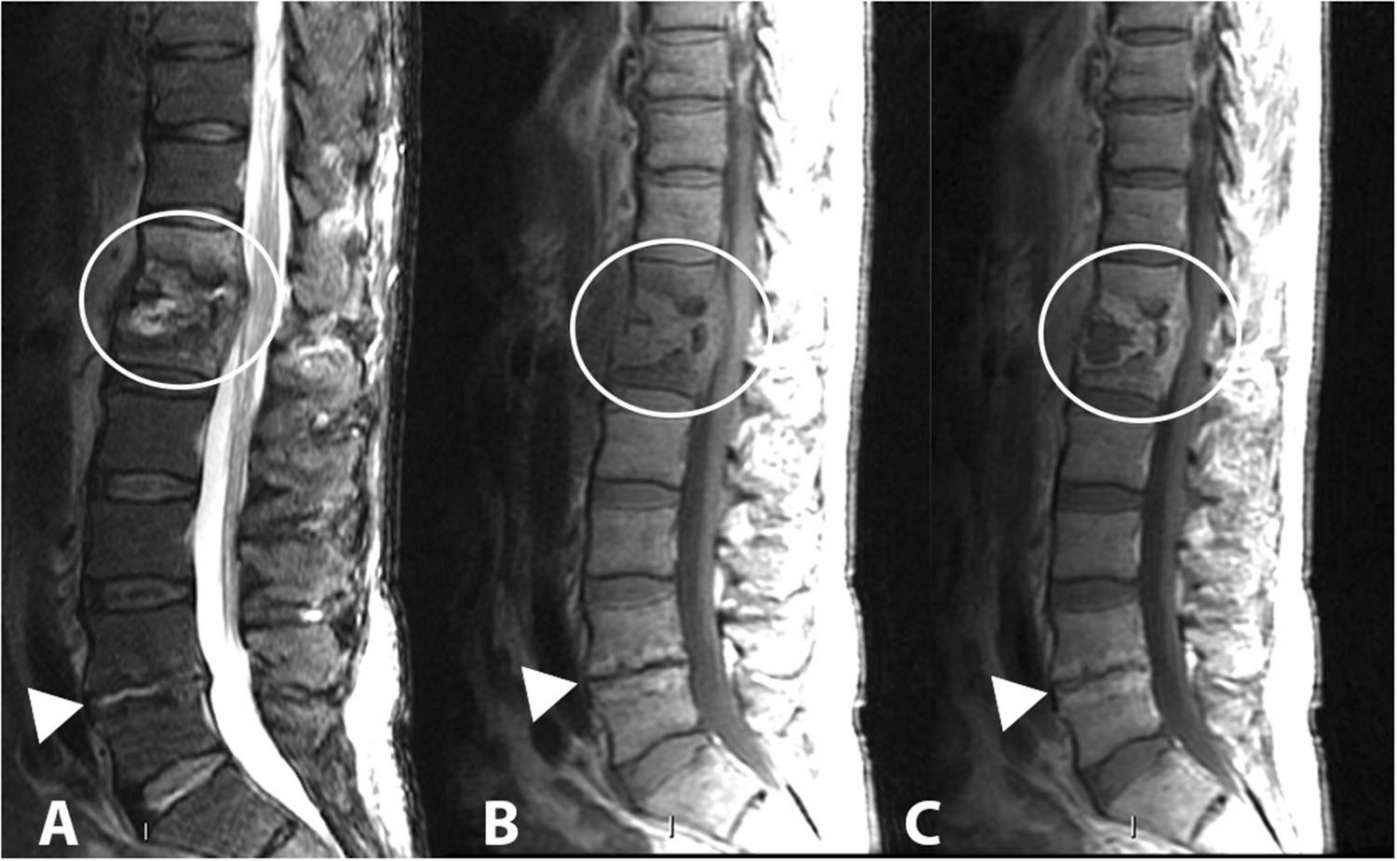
Spondylodiscitis caused by Staphylococcus aureus. The D12-L1 disc and the adjacent vertebral bodies appear markedly involved in the infectious process. The fat saturated T2-weighted sequence (a) and the sagittal T1-weighted sequence (b) show an altered signal intensity of the affected segments. The sagittal T1-weighted image after gadolinium administration (c) highlights a significant contrast enhancement of the affected area, with a posterior extension into the epidural space (white circle). In addition, a Modic 2 lesion can be observed at L4/L5, with hypointense signal in STIR image (a) and high signal intensity in T1-weighted images (b, c) (arrowhead)
